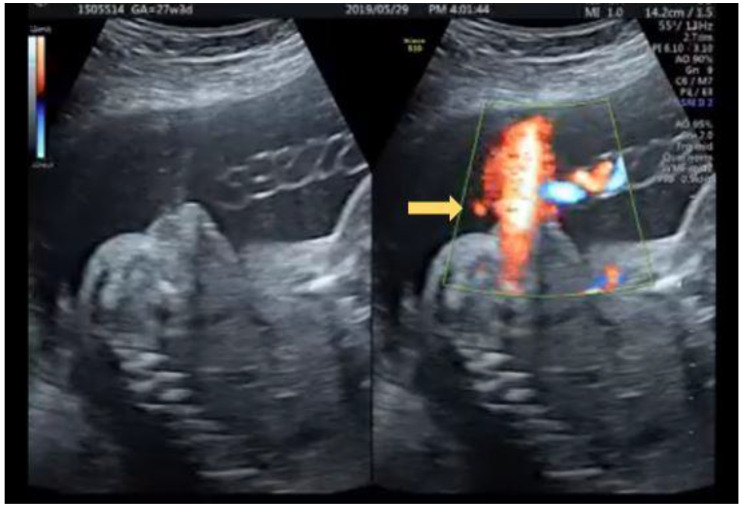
Fanning of urinary stream originated proximal to perineum under color Doppler (arrow).
